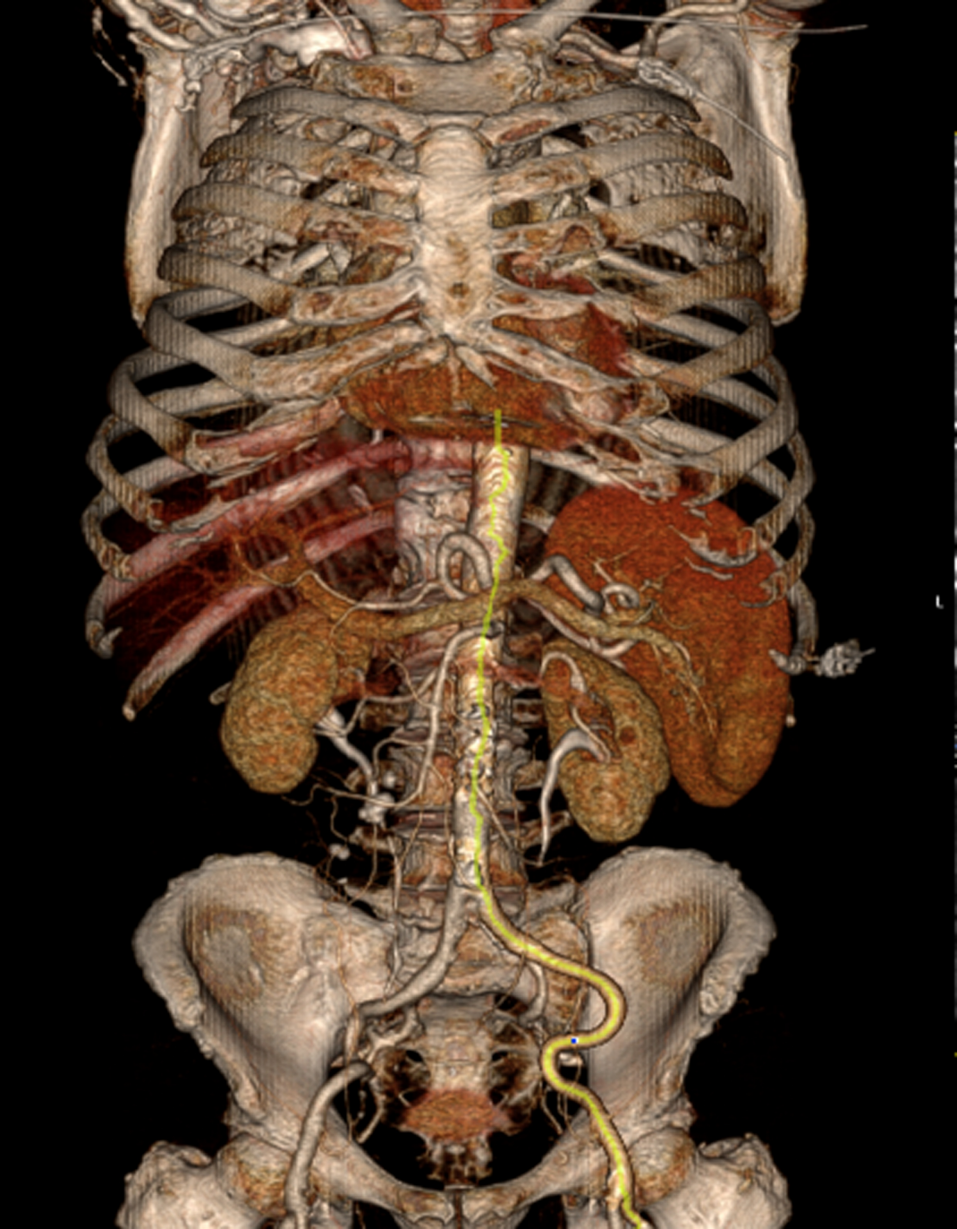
Multislice CT angiography presents iliofemoral arterial segment - suitable for transfemoral approach. CT - computed tomography
